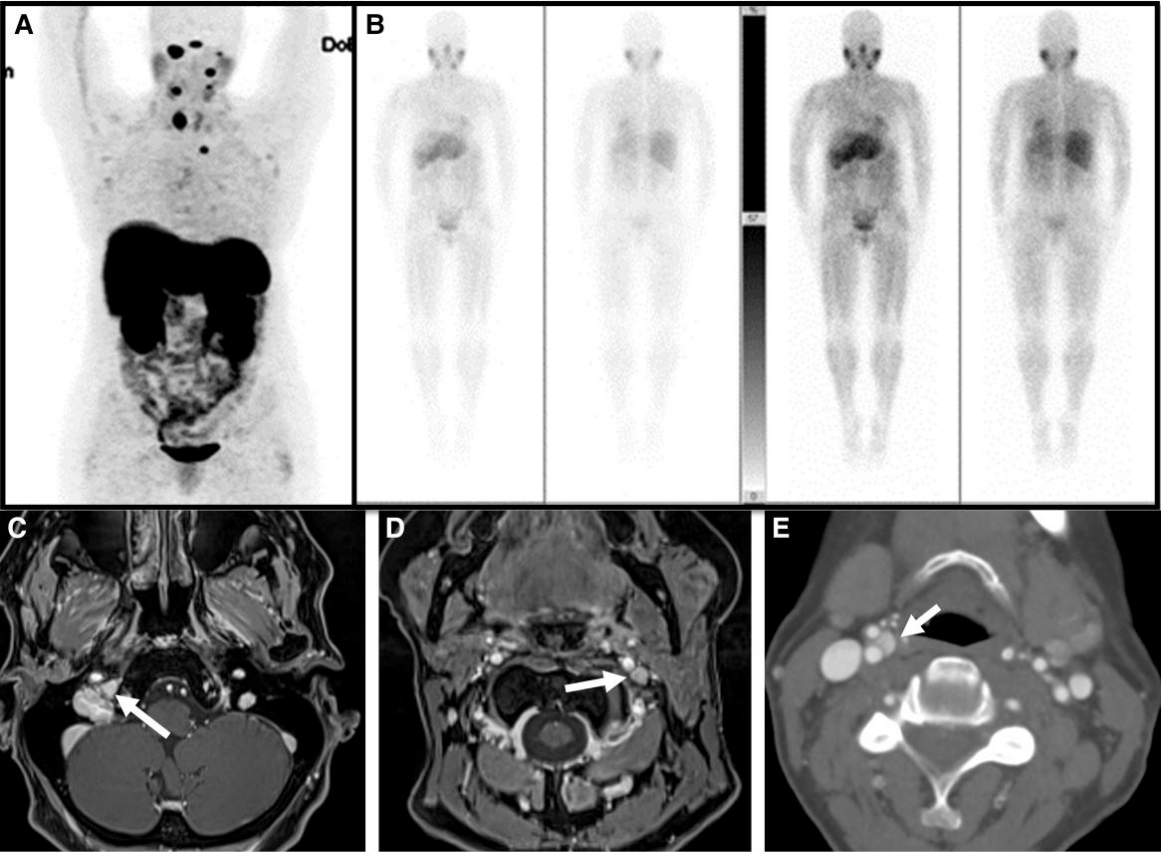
Images of multiple head and neck paragangliomas. A: Gallium-68 (68Ga)-DOTATATE positron emission tomography/computed tomography (PET/CT) revealed multifocal areas of increased somatostatin receptor expression from the skull base to the left thoracic inlet (right and left skull base, left level IIA, right and left level III, right thyroid lobe, and left prevascular superior mediastinum). B: Metaiodobenzylguanidine (MIBG) nuclear medicine scan revealed no radiotracer accumulation in the lesions. C, D, E: Magnetic resonance imaging (MRI) of the neck with and without contrast showed right jugular skull base 1.7 × 1.2 cm paraganglioma (C), 0.6 × 0.5 cm left vagal paraganglioma (D), and 0.6 × 0.6 cm right carotid paraganglioma (E).
